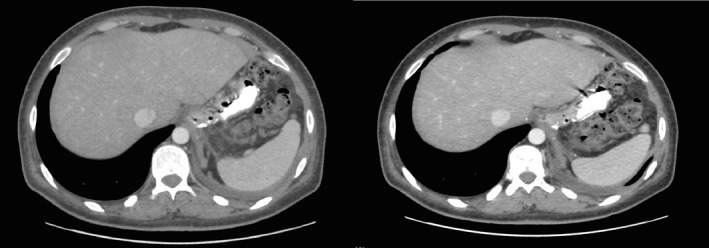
CT scan abdomen with PO contrast showing resolution of the gastro‐colic fistula showing continuous staple line with no extravasation of contrast.
